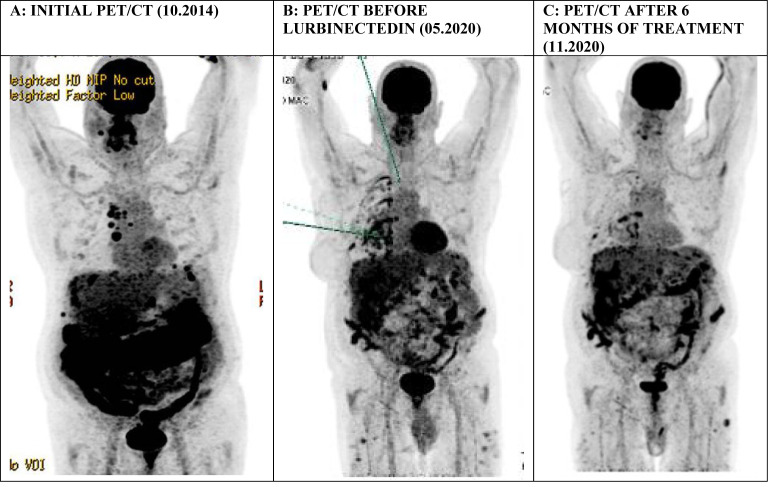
A series of PET/CT scans (Maximum Intensity Projections) in the first patient showing: (A) signs of peritoneal carcinomatosis in the subdiaphragmatic space infiltrating the large omentum and associated with ascites, in addition to supradiaphragmatic laterocervical and mediastinal lymphadenopathies, (B) persisting multiple peritoneal implant lesions at the time of treatment initiation, (C)  a significant improvement of the previously described peritoneal lesions with an overall regression of their metabolic activity.
